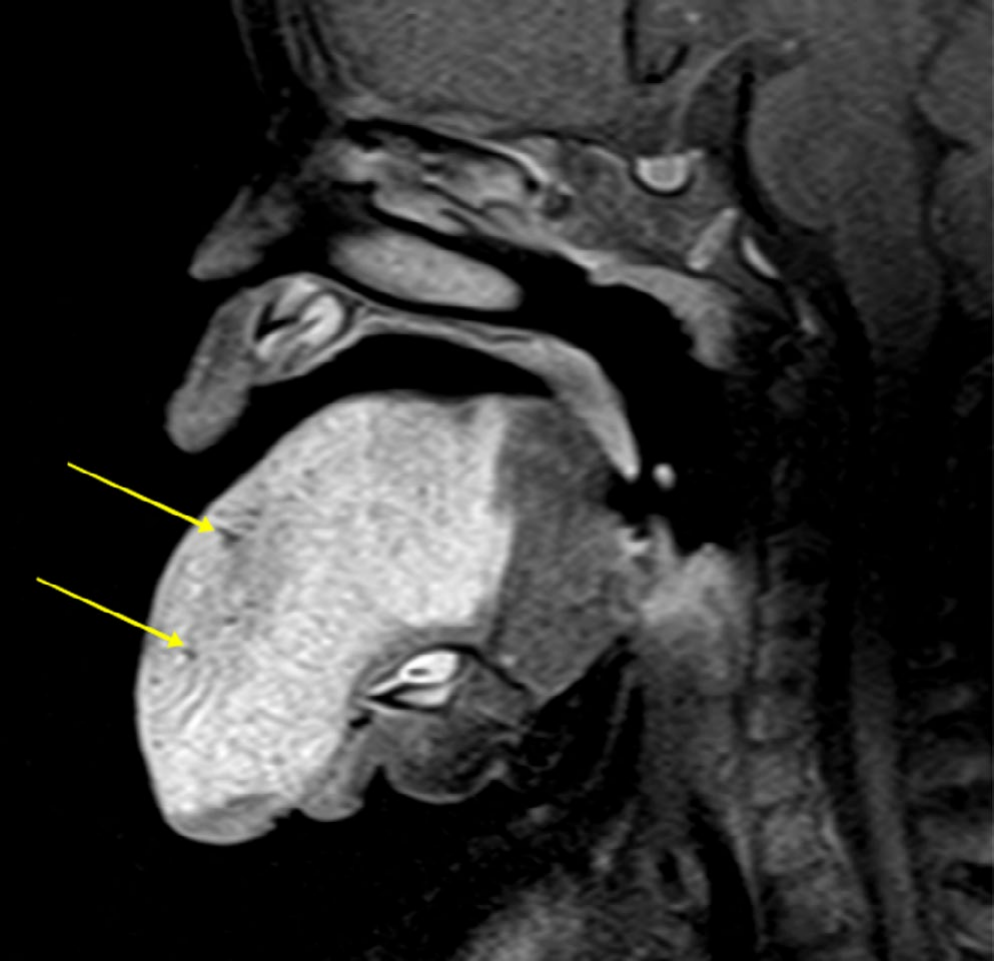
T2‐weighted contrast enhanced MRI sagittal section showing a hyperintense mass on the tongue. Small foci (yellow arrows) can be seen within the lesion corresponding to flow voids
